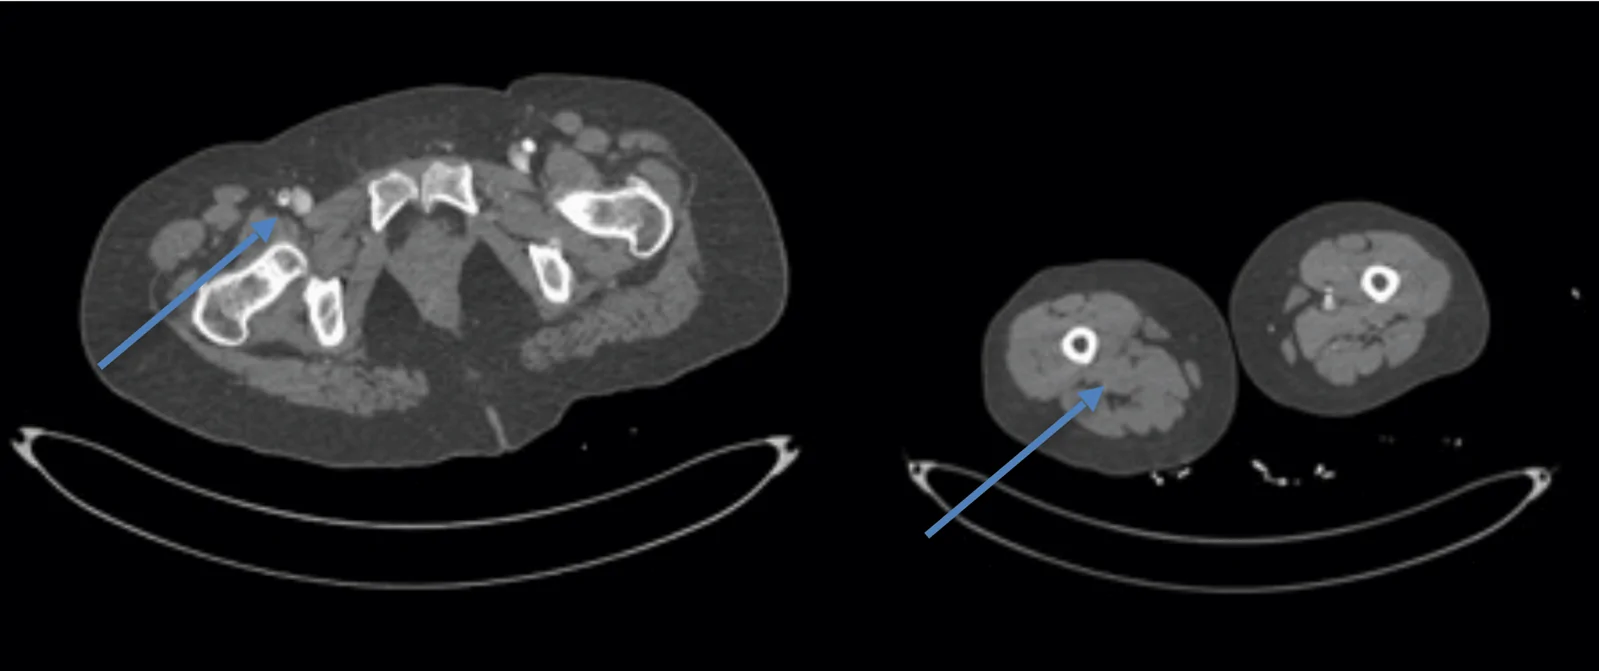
CT angiography of the lower limbs demonstrating bilateral acute limb ischemia Axial and coronal images showing long-segment occlusions of the right superficial femoral, popliteal, anterior tibial, and posterior tibial arteries (arrows), with markedly reduced distal perfusion bilaterally.
